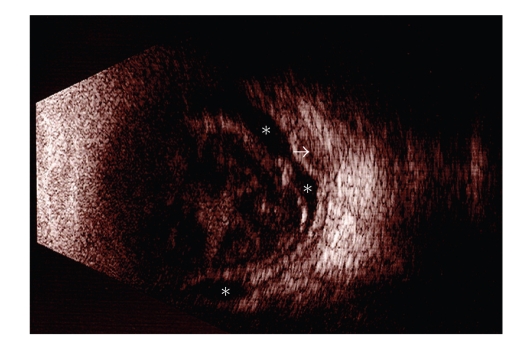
Right eye ultrasound B-scan with both retinal (asterisks) and choroidal (arrow) detachments.
